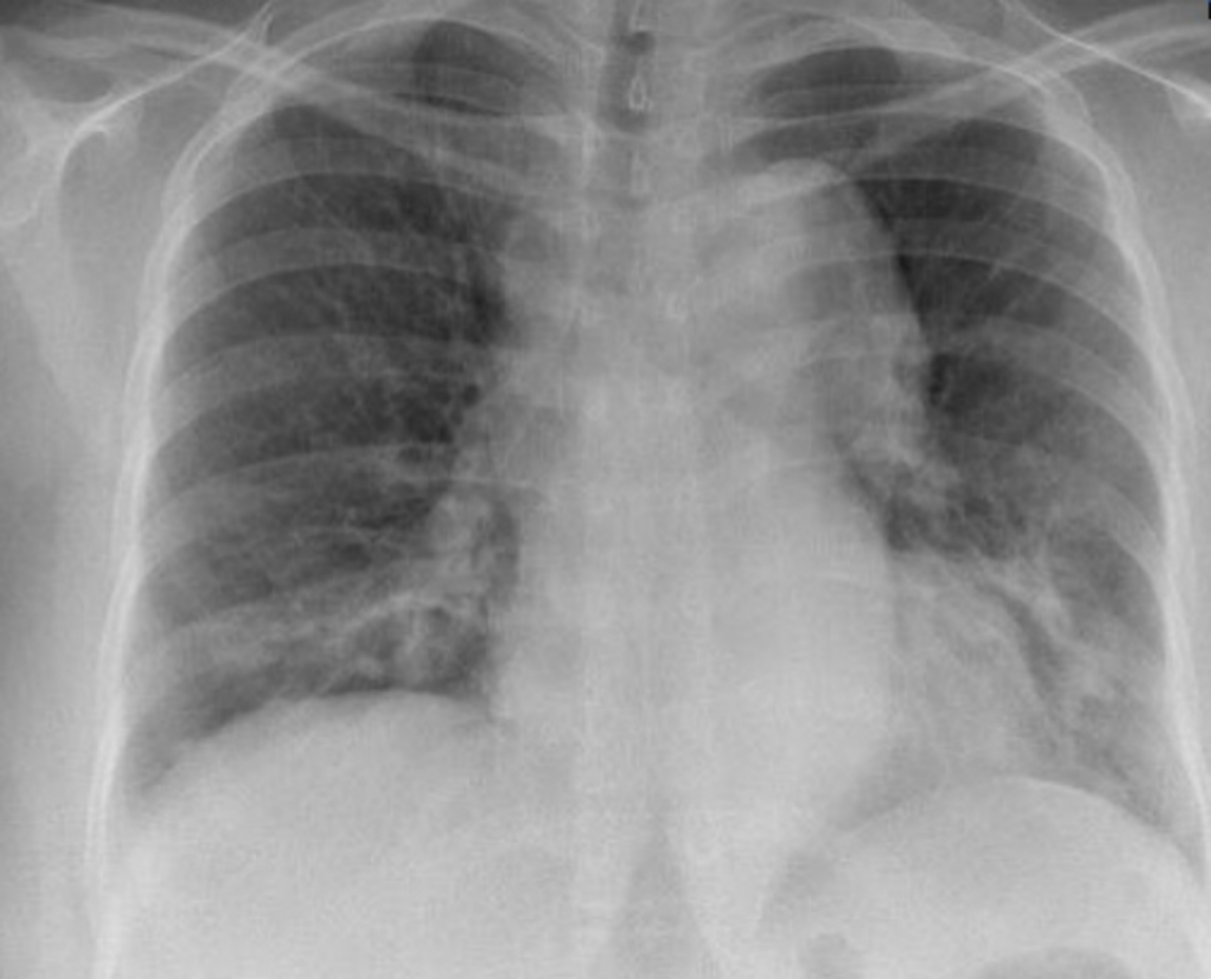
X-ray showing widened mediastinum, 2014.
